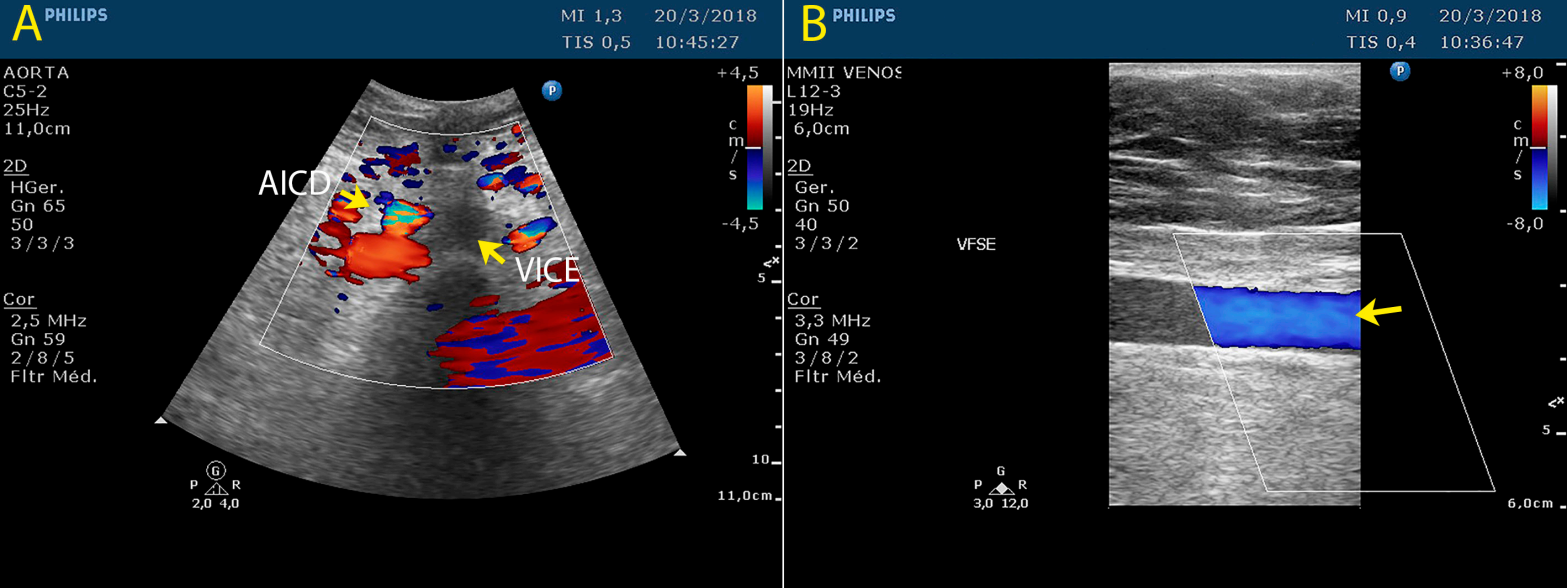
Duplex ultrasound (DUS) showing: (A) left common iliac vein (LCIV) with large caliber and no blood flow, with hypoechoic intraluminal material indicating acute deep venous thrombosis (DVT) and compression by the right common iliac artery (RCIA); and (B) left superficial femoral vein (LSFV) with normal caliber, flow present, and absence of thrombi.
